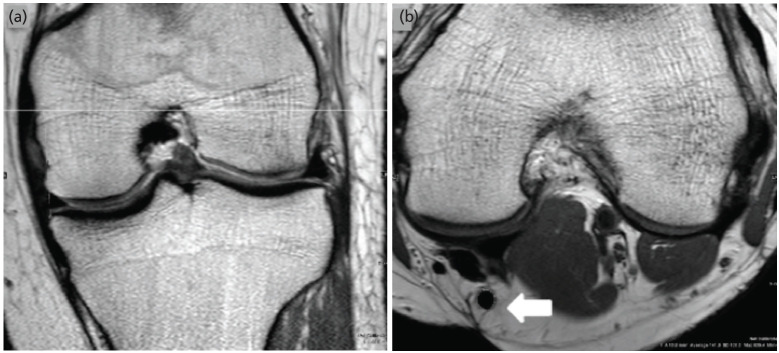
MRI coronal and sagittal views showing the method of estimation of the cross-sectional area of Semitendinosus tendon. The corresponding (a) PD coronal and (b) PD axial section showing the cross-section of Semitendinosus (white solid arrow) at the maximum tendon diameter was recorded using the ROI tool.
